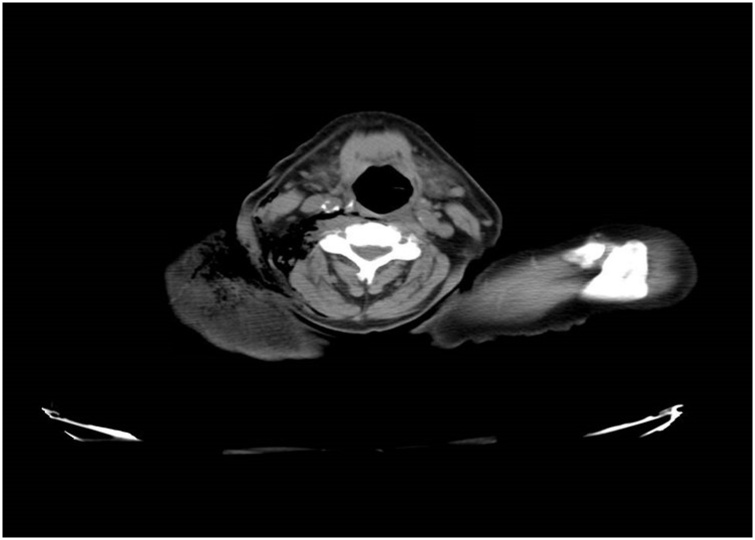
CT scan neck showing air in the soft tissues around right side of the neck.
